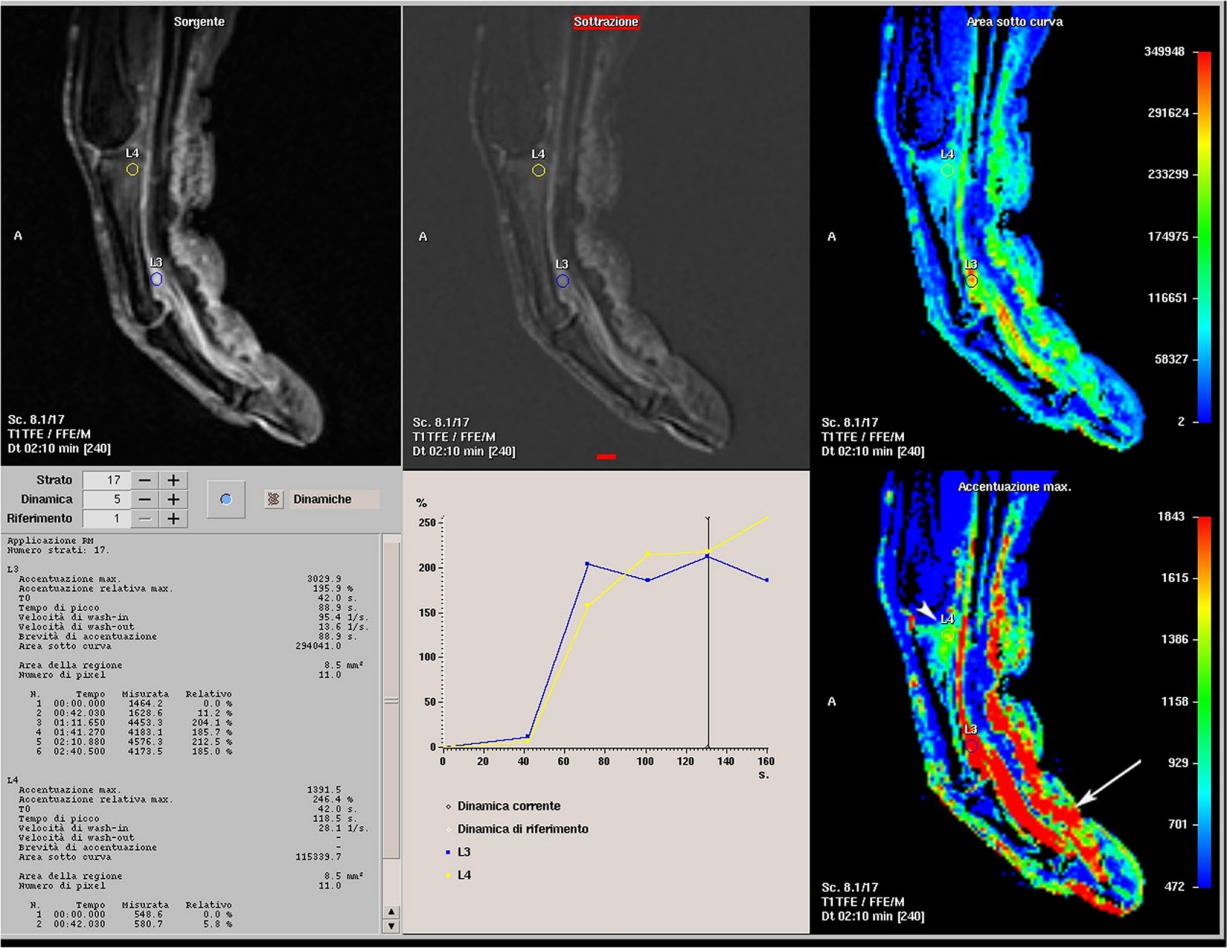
Sagittal T1-weighted sagittal image with fat saturation obtained during the course of the contrast medium uptake of a dactylitis finger of a 59-year-old man. There is enhancement of the flexor tendon synovial sheaths (arrow) and of a functional enthesis at the base of the proximal phalange (arrowhead)
